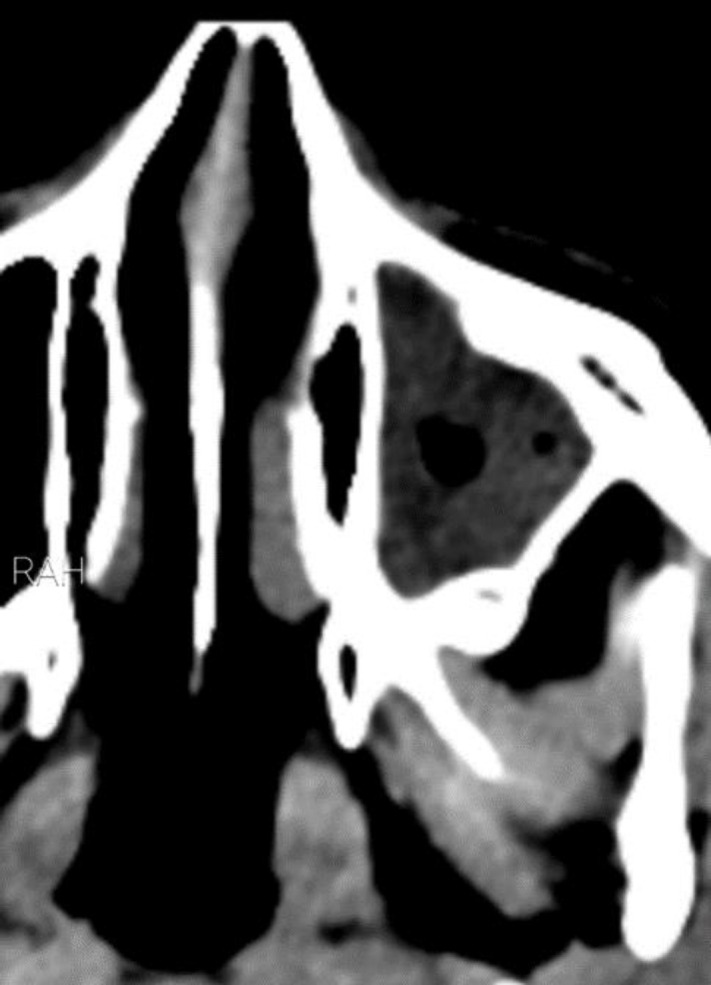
Contrast enhanced computed tomographic scan of head showed right maxillary sinus opacification and right nose fullness
